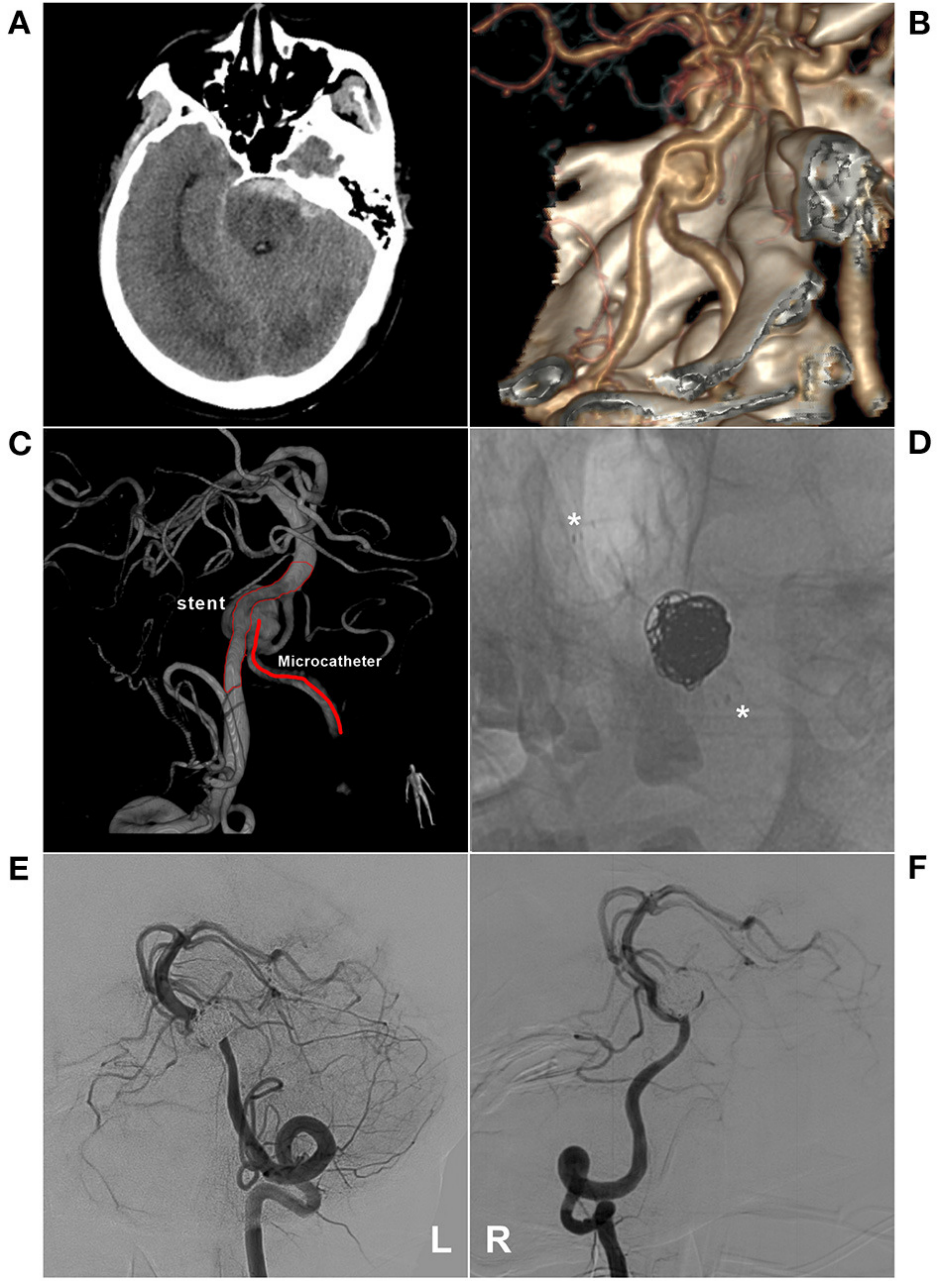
EVT with stent-assisted coiling of an aneurysm in the fenestration. (A) CT shows subarachnoid hemorrhage at the front of the brainstem; (B) CTA shows an aneurysm in a fenestration of the inferior BA. (C) 3D-reconstructed DSA shows the aneurysm and EVT schemes. The stent was placed into a coarse VA and microcatheter-walked to a finer VA to embolize the aneurysm; (D) X-ray image shows coiling with stent-assisted EVT (asterisk); E-F: VA-DSA on the left (E) and right (F) side show the aneurysm embolization, MRRC Grade IIIa. BA, basilar artery; CT, computed tomography; CTA, CT angiography; DSA, digital subtraction angiography; EVT, endovascular therapy; MRRC, Modified Raymond-Roy Classification; VA, vertebral artery.
